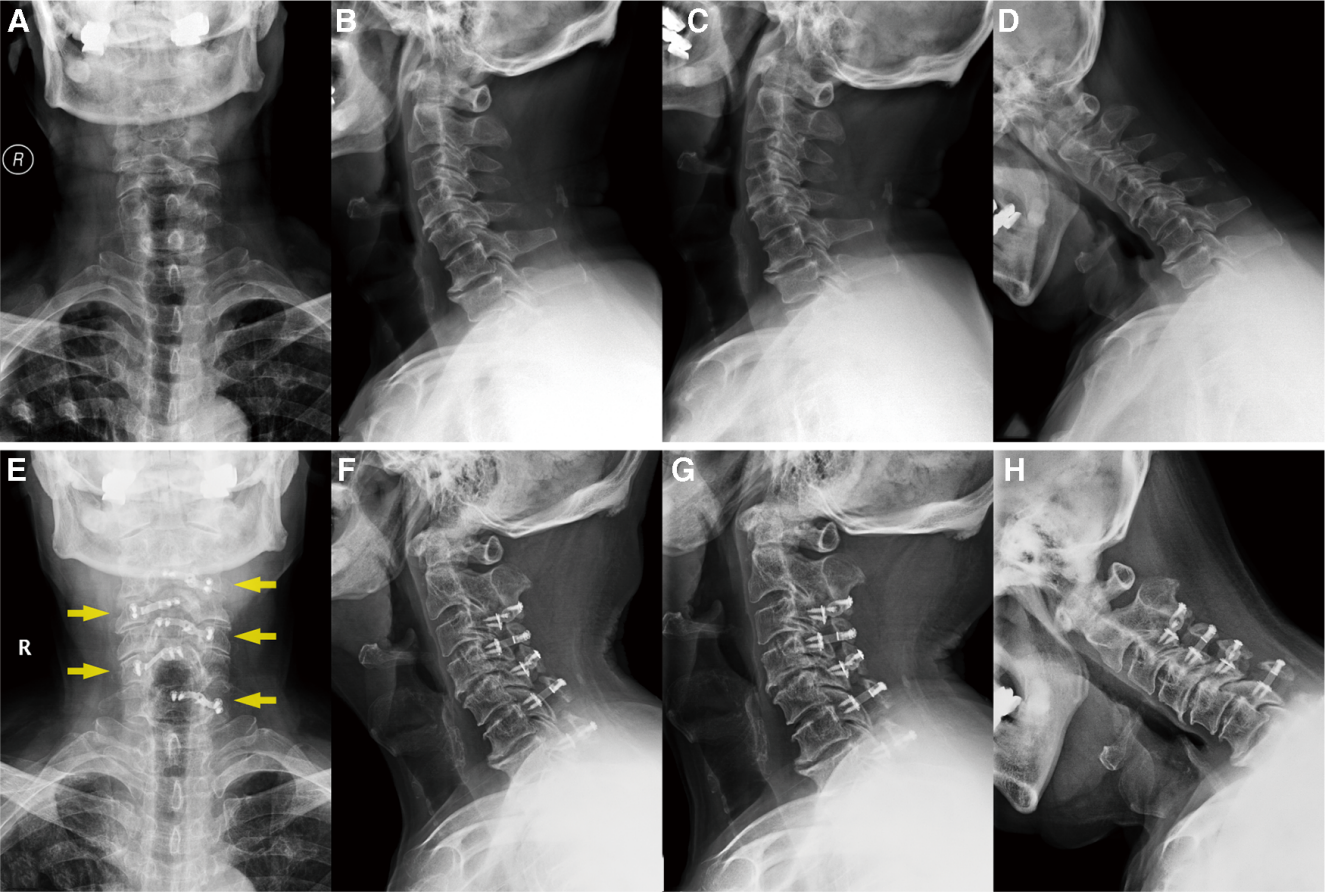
(A–D) Preoperative x-rays of the cervical spine (anteroposterior, lateral, hyperextension, and flexion radiographs, respectively), showing that the vertebral physiological curvature straightened with a certain degree of degenerative change. (E–H) Postoperative x-ray after 5 years, demonstrating the position of the internal fixation plates (yellow arrows) and that the cervical curvature and the range of motion have remained the same.
